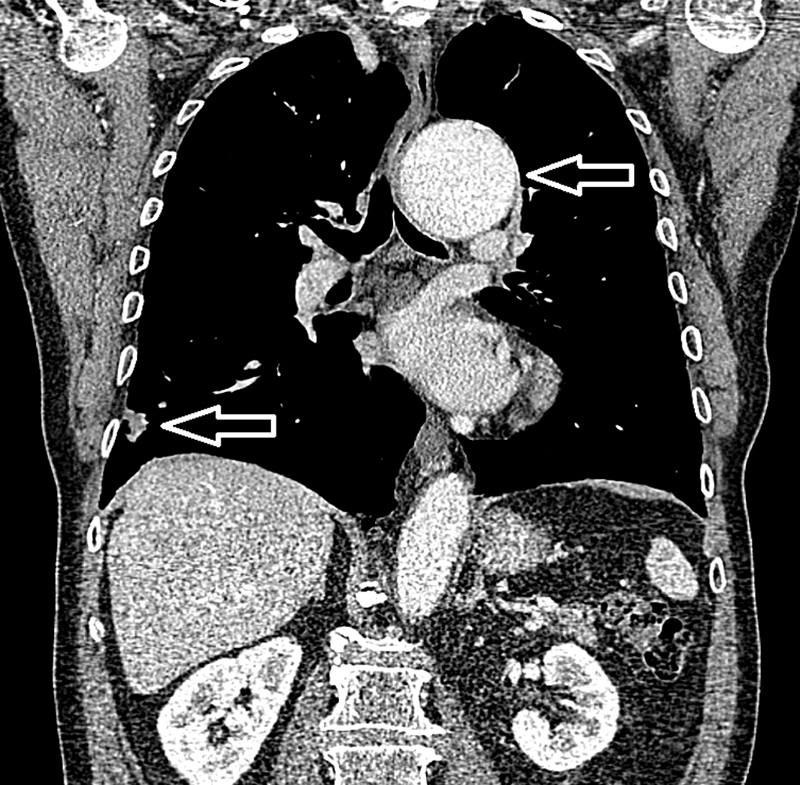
Preoperative computed tomography (CT) demonstrating proximal descending aorta dilation (top arrow) and right lower lobe lesion (bottom arrow). It was identified in the preoperative CT that the patient had both a dilated aortic root and postcoarctation dilation extending to the proximal descending aorta.
